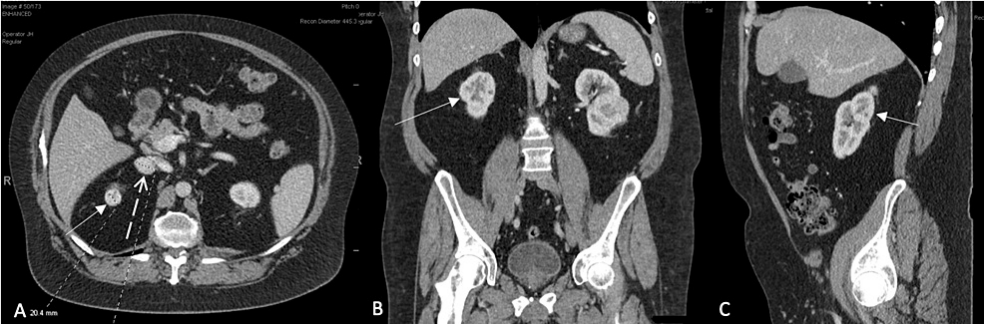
Right renal mass on contrast CT. The solid white arrows indicate the right renal mass in the transverse (2A), coronal (2B), and sagittal plane (2C), and the dashed arrow (2A) indicates the left adrenal mass.
